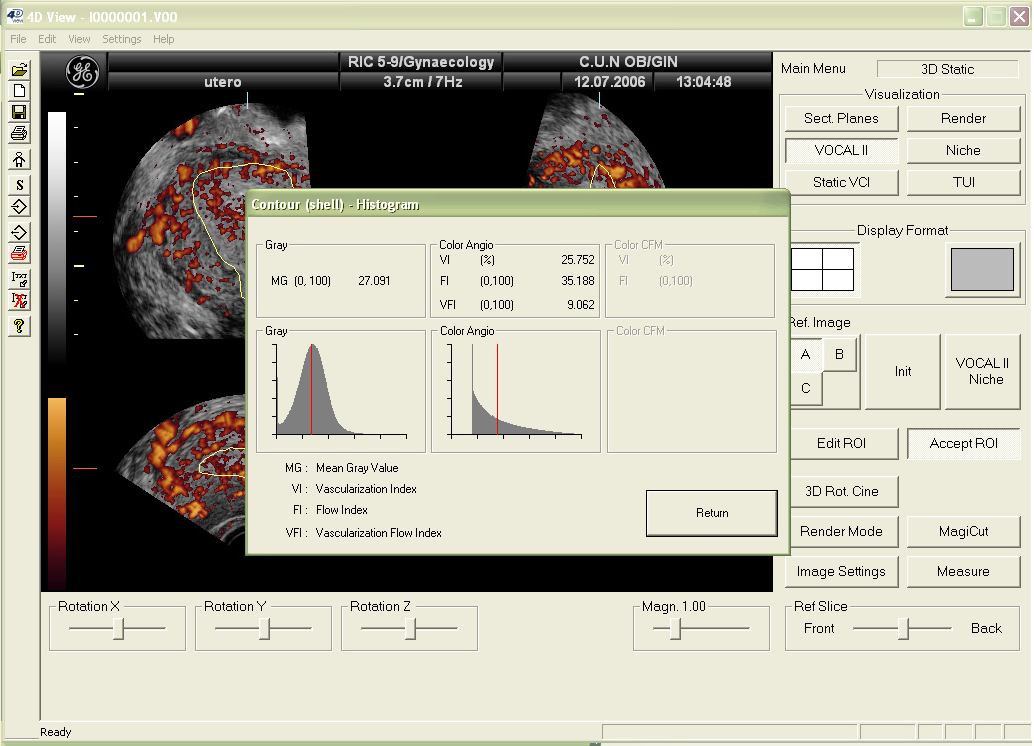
3D-Power Doppler indexes for assessing endometrial vascularization by means of the three-dimensional ultrasound.
